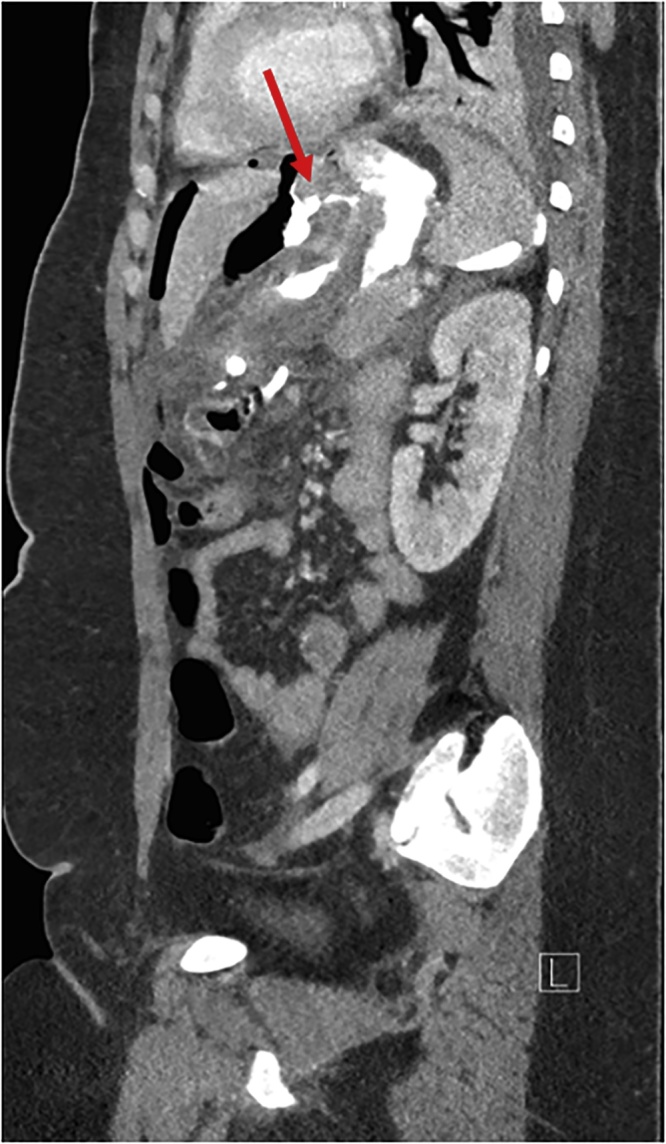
One day after laparoscopic gastric banding conversion to sleeve gastrectomy: sagittal plane of CT scan with obvious anterior cardial leak (red arrow).
